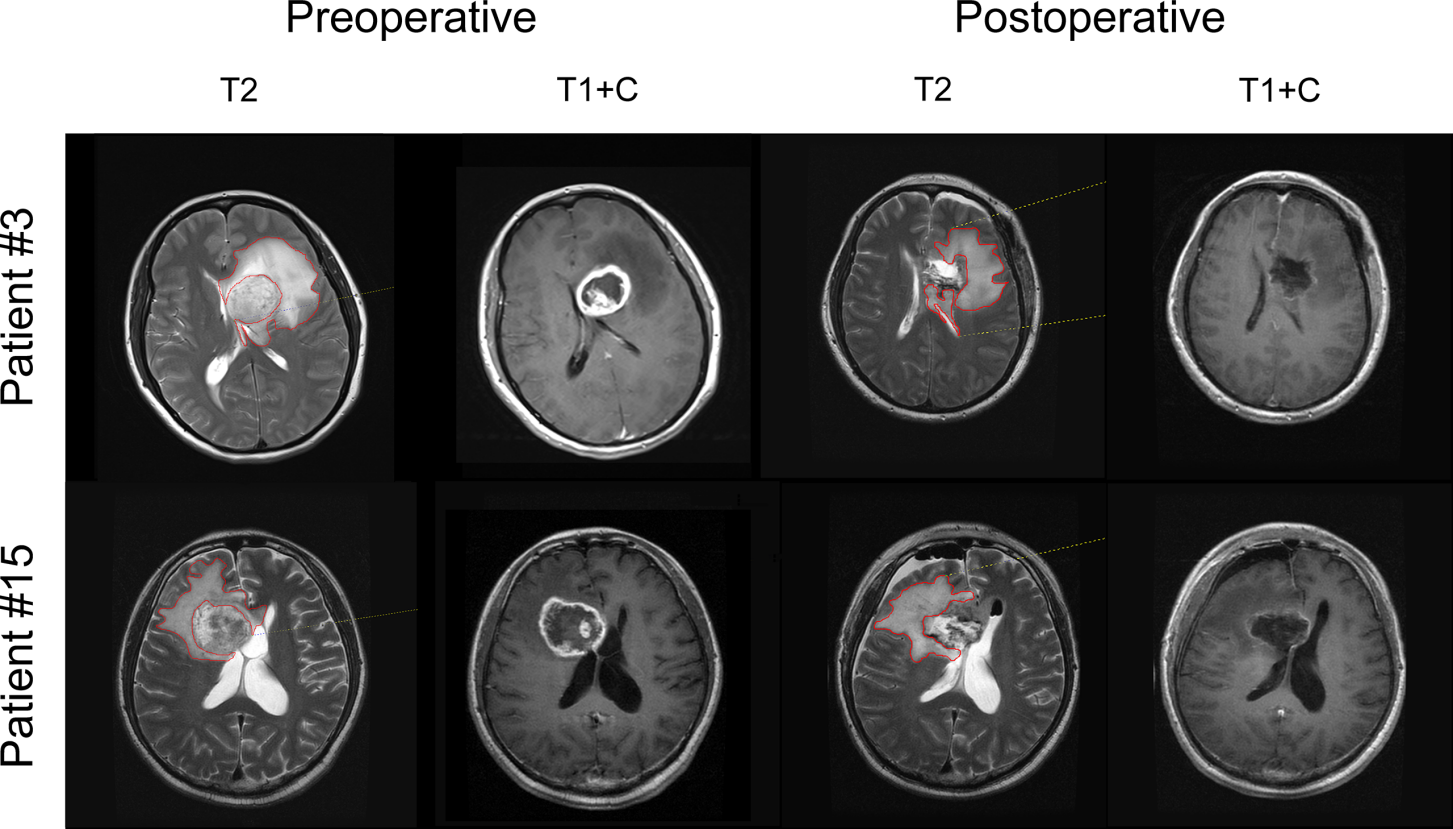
T2-EOR determination on preoperative and postoperative MRI images. The noncontrast-enhancing regions were represented as T2-weighted hyperintense areas outside the T1-weighted contrast-enhancing lesions and were termed T2-nonenhancing regions. T2-nonenhancing regions were manually outlined in red at each level, and the volume was automatically calculated by Neusoft PACS software. T2-EOR was defined as the preoperative-to-postoperative change in T2-nonenhancing region volume divided by the preoperative T2-nonenhancing region volume.
